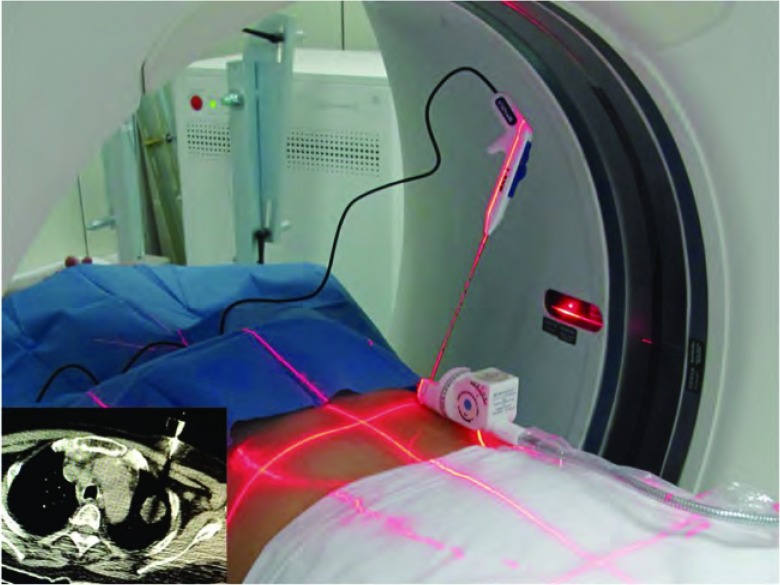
导向器辅助的CT引导穿刺：将CT激光定位线与导向器支撑的穿刺针完全重合，CT扫描可见穿刺针的延长线或者尾影通过靶点（左下图），迅速穿刺至相应深度，即可命中靶点 A directive apparatus assisted CT guided puncture method: overlap the CT laser light with puncture needle, CT scan shows an extension cord or shadow tail of needle through the target (lower left), then puncture to the depths rapidly to targeting the lesion. CT: computed tomography
